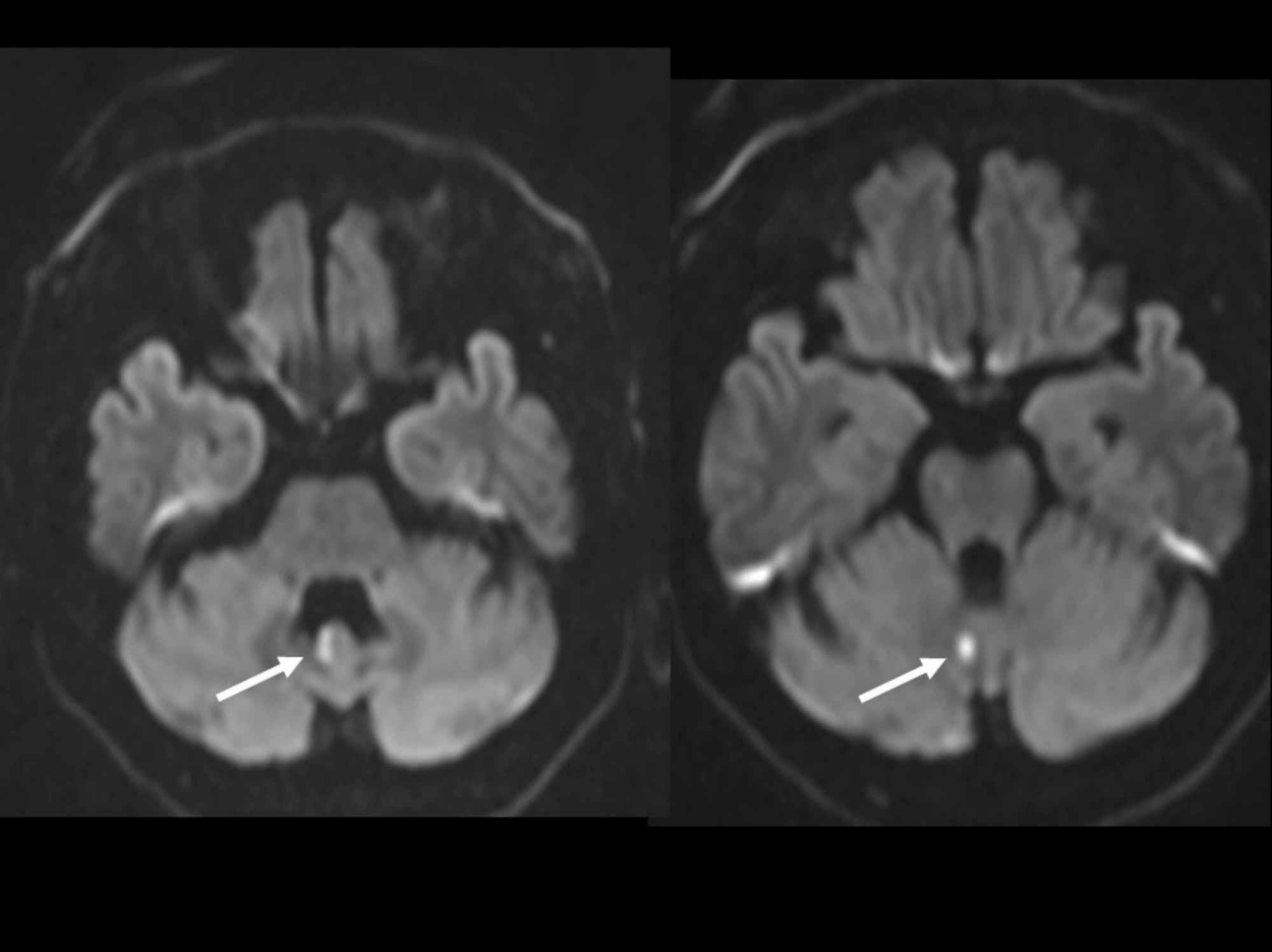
Diffusion-weighted scan of brain magnetic resonance imaging (MRI) on the second hospital day. MRI revealed cerebellar infarction (arrow).
